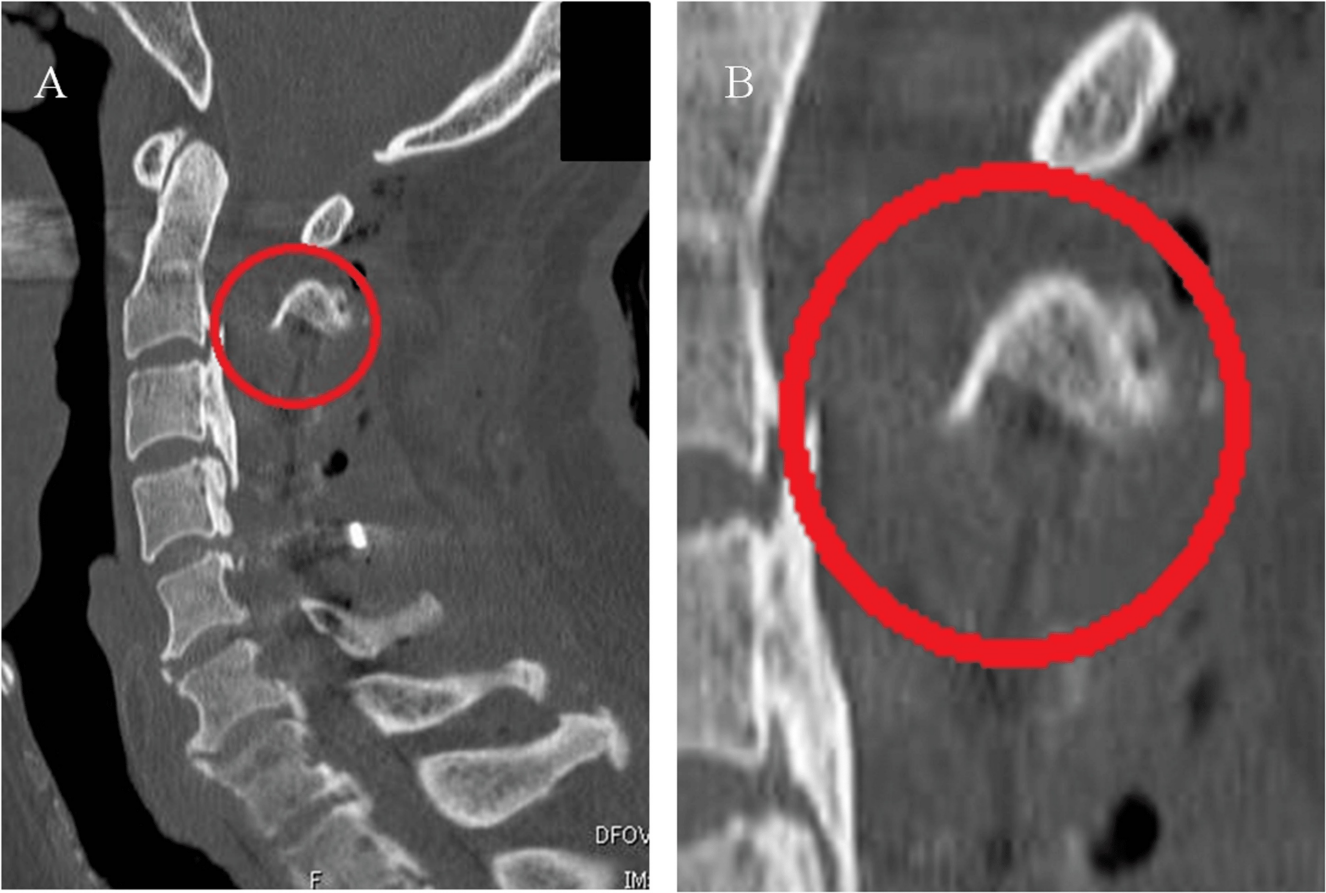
CT images of the cervical spine taken the day after surgery (A) Midsagittal section; (B) Magnified image. C2 dome-like laminectomy was performed on the C2 lamina. The residual edge (ovals) at the base of the C2 lamina were observed, which may have contributed to dural damage, as their location coincided with the site of spinal cord herniation.
